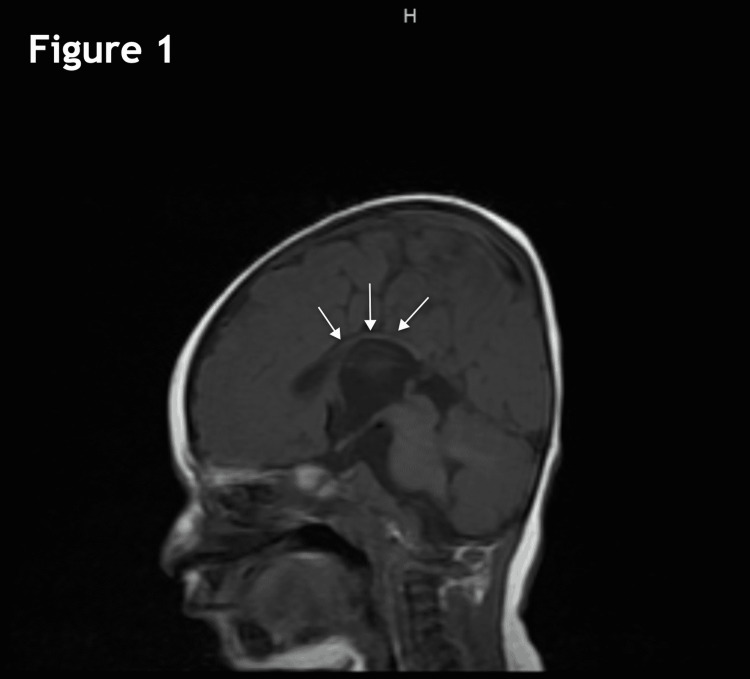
Sagittal T1-weighted image showing marked thinning of the corpus callosum with possible partial dysgenesis
